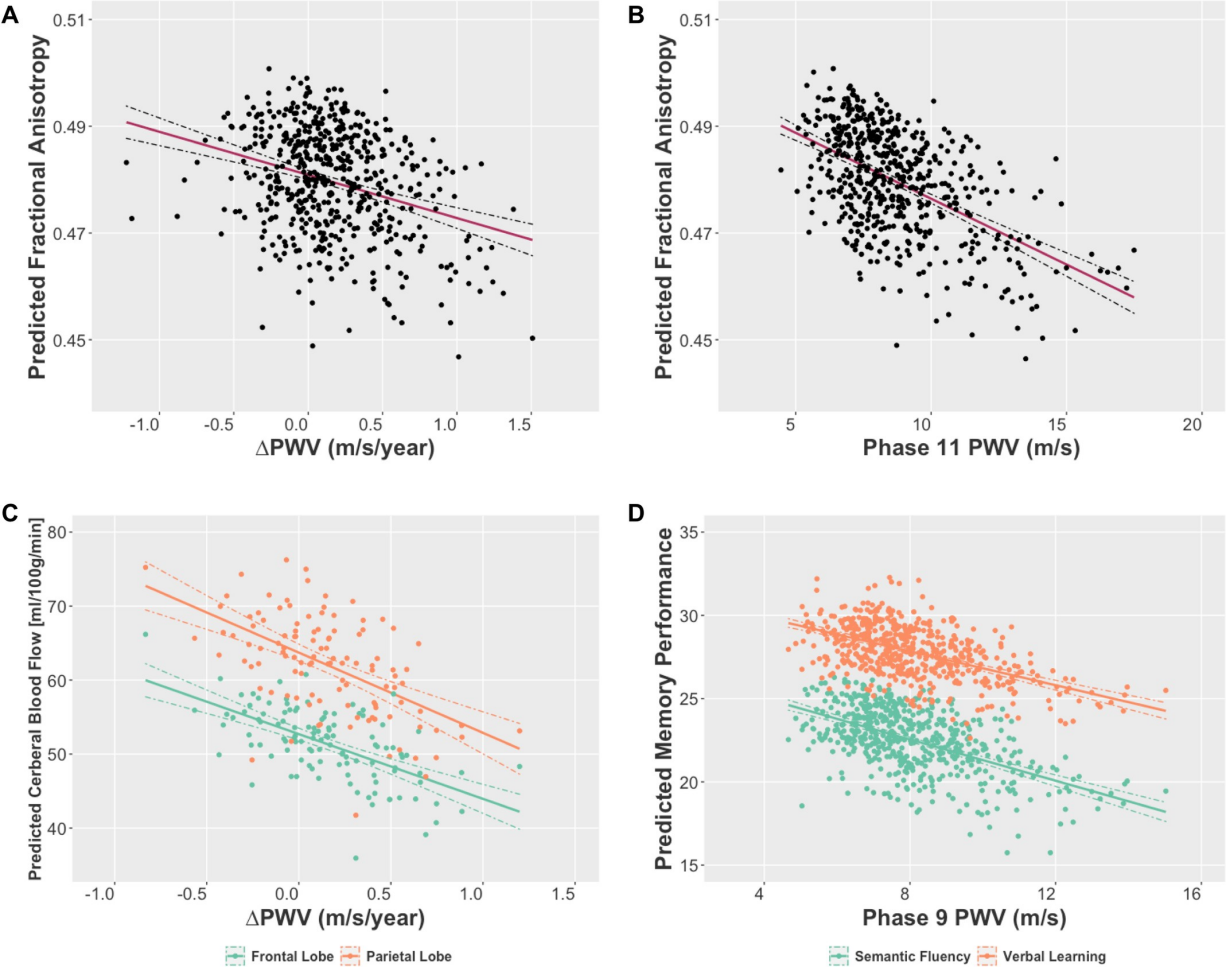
Partial regression plots showing the associations of PWV with brain and cognitive outcomes which survived Bonferroni correction for multiple comparisons. Plots present estimated marginal means for (A, B) Predicted global FA, (C) Predicted CBF from frontal (green) and parietal (orange) lobes, and (D) Predicted memory performance on semantic fluency (green) and verbal learning (orange) tests, plotted against (A,C) Rate of change of PWV, (B) PWV at Phase 11, and (D) PWV at Phase 9. Estimated marginal means are derived from linear regression models adjusted for age, MAP, BMI, and antihypertensive treatment measured at the respective phase (9 or 11), number of years from the respective phase (9 or 11) to the MRI scan, and sex, education, socioeconomic status, and MRI scanner model. BMI, body mass index; CBF, cerebral blood flow; FA, fractional anisotropy; MAP, mean arterial pressure; MRI, magnetic resonance imaging; PWV, pulse wave velocity.
